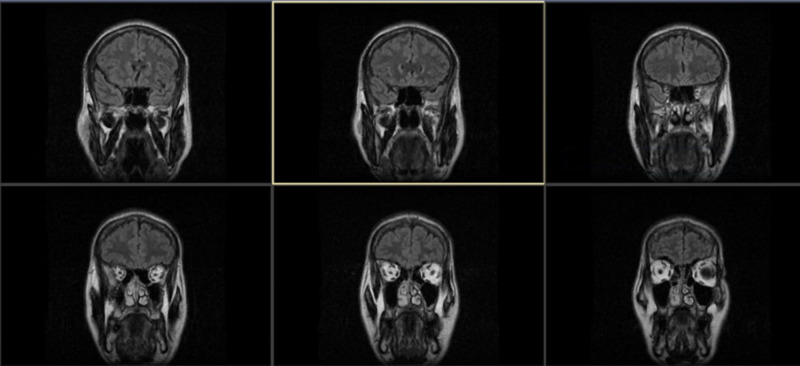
Brain MRI with and without contrast
